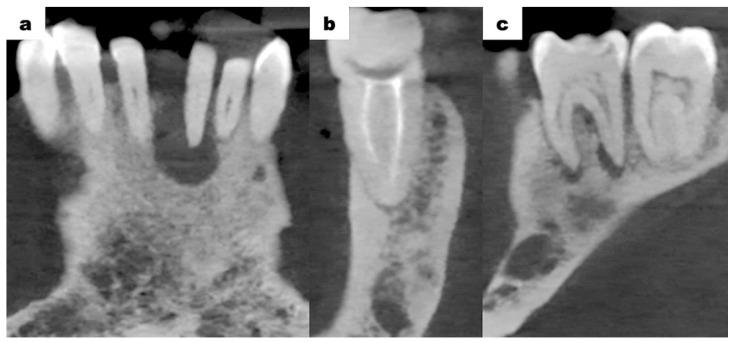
CBCT examination scans before initiation of treatment; (a) tooth 31—inflammatory change involving the entire root; (b) tooth 46—no trace of filling material in the root canals in the apical region, a suspected periapical lesion; (c) tooth 36—periapical inflammatory changes visible around both roots.
